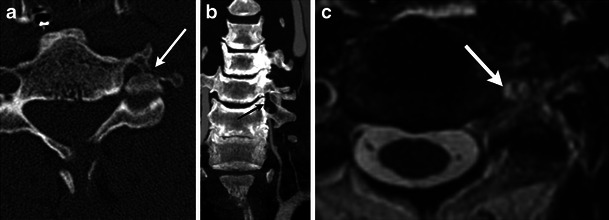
(a) Axial MDCT image shows the fracture extending through the foramen transversarium (white arrow). (b) Coronal CT angiogram MIP image demonstrates sudden cutoff of the left vertebral artery consistent with dissection (black arrow), which is also seen on the axial T2-weighted image (c) as loss of flow void (white arrow)
